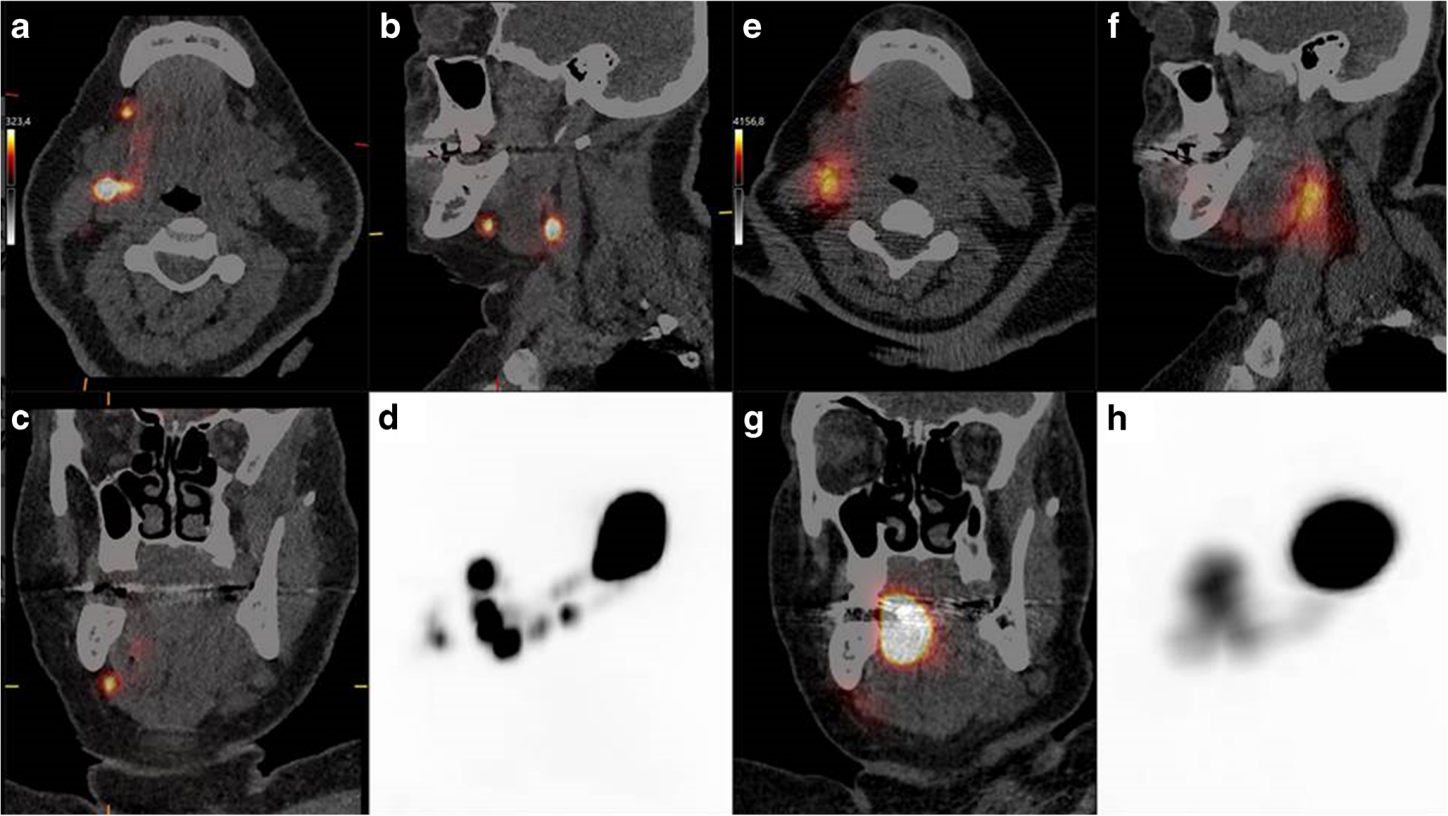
P[^68^Ga]Ga-tilmanocept PET/CT vs. [^99m^Tc]Tc-tilmanocept PET/CT.
